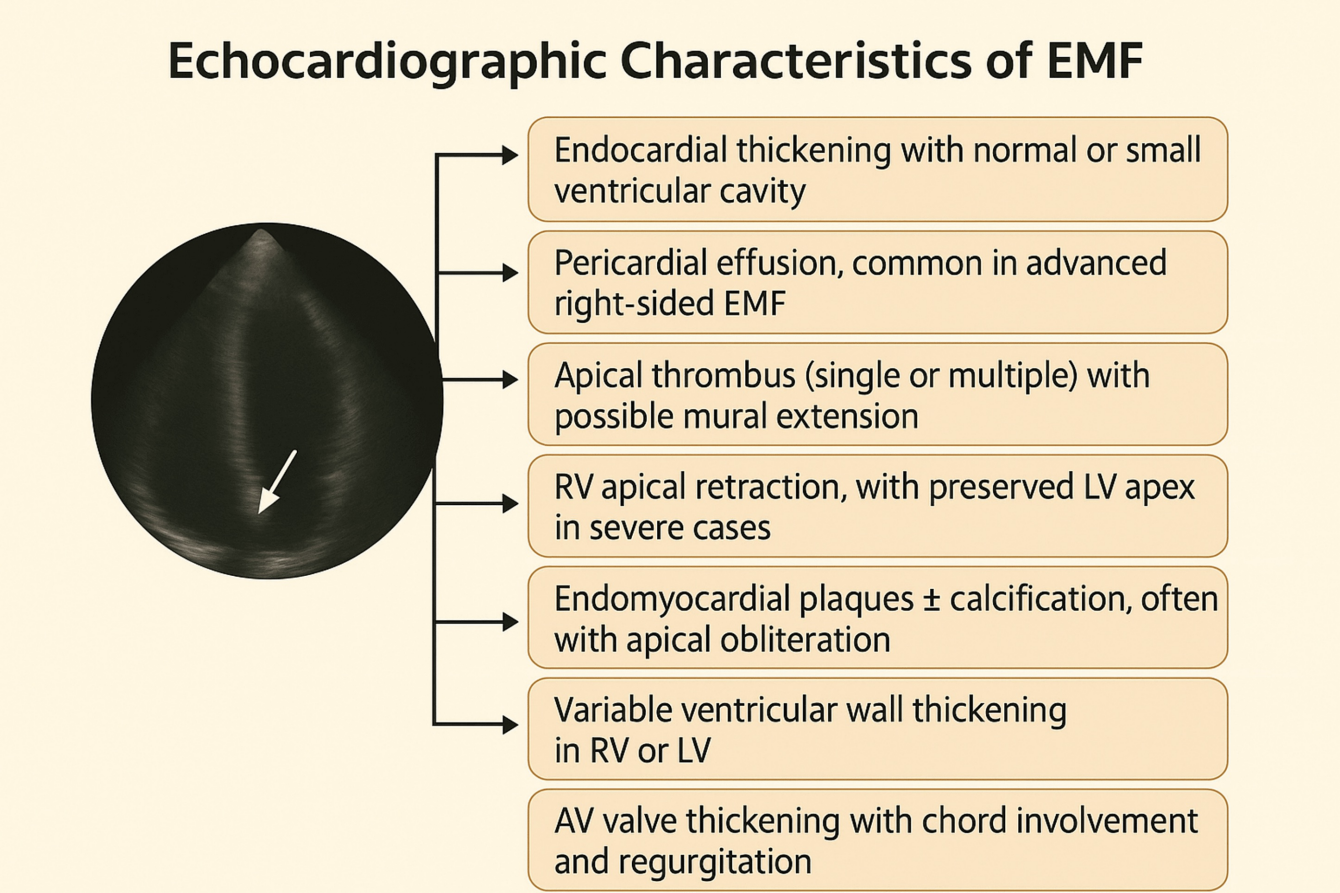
Echocardiographic characteristics of EMF EMF: endomyocardial fibrosis; LV: left ventricular; RV: right ventricular; AV: atrioventricular Credits: Sri Lakshmi Kothakapa, created with biorender.com
